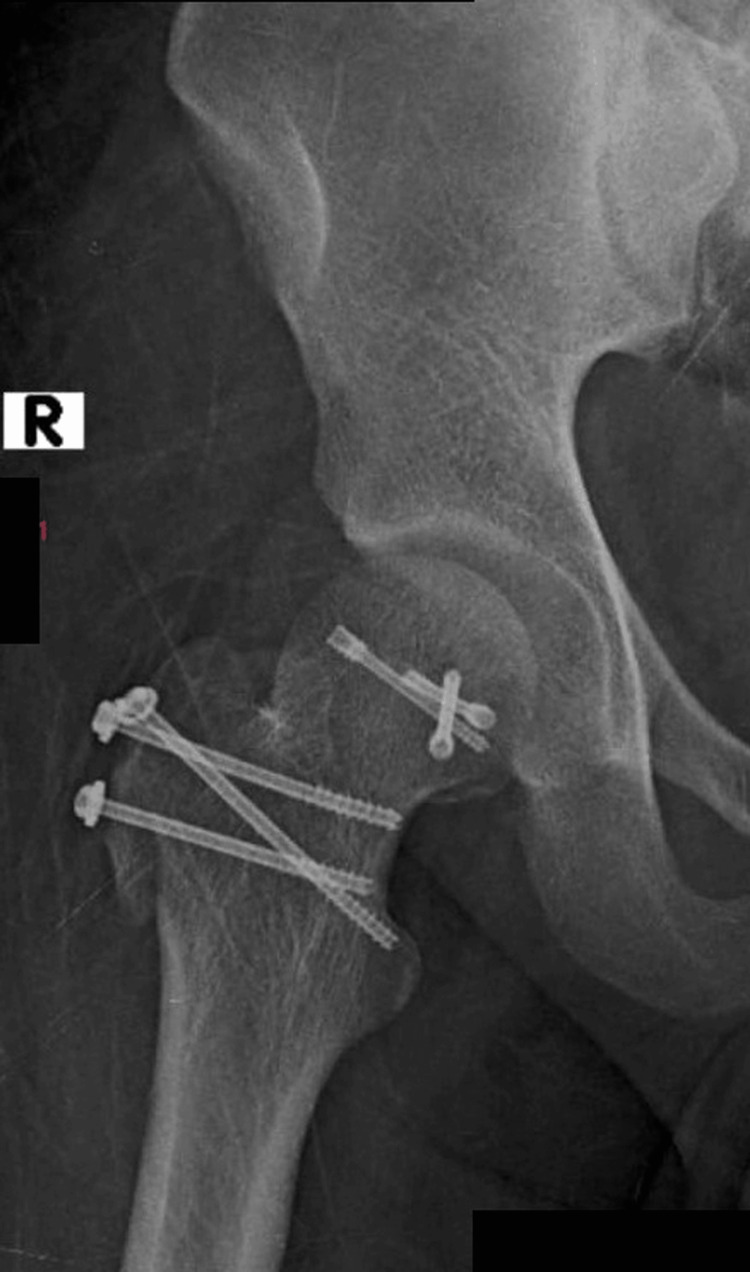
Four-week post-operative follow-up anteroposterior radiograph of the right hip. The radiograph demonstrates well-maintained anatomical reduction of the femoral head fragment with three countersunk headless compression screws, anatomical position of the greater trochanteric flip osteotomy held by three 3.5 mm cortical screws, a concentric hip joint, preserved joint space, no femoral head collapse, and no heterotopic ossification.
